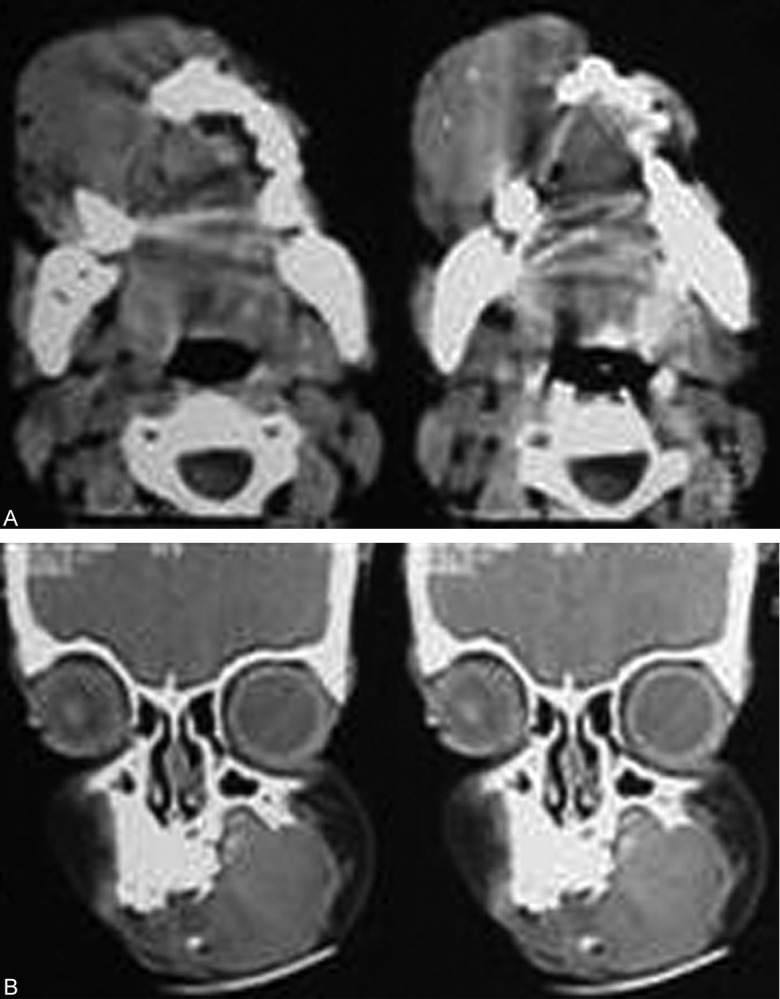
CT scans showing an expansile lesion eroding the buccal cortical plate of right maxilla
